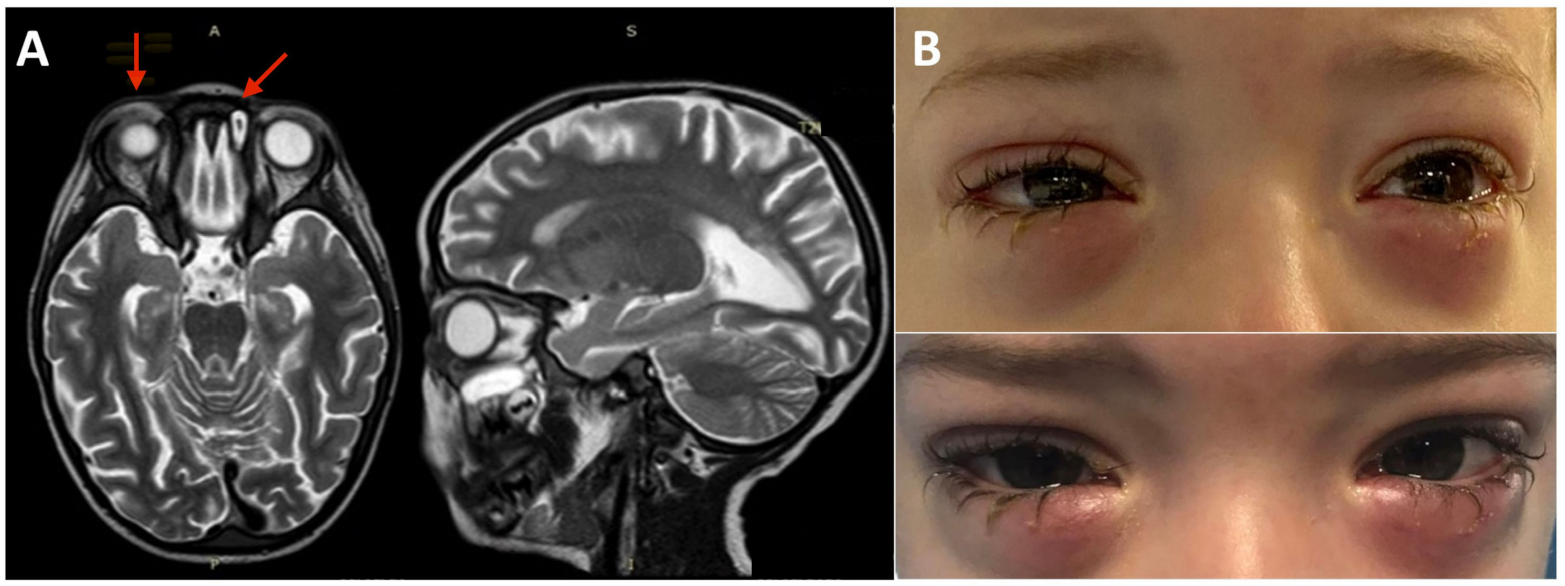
Brain, eye orbit, and paranasal sinus MRI and bilateral lower eyelid edema. (A) MRI of brain, eye orbits, and paranasal sinuses showing bilateral obliteration of the ethmoid-maxillary sinuses, an inflammatory thickening of the periorbital subcutaneous adipose tissue, hypointense in T2 in both orbits, but more evident in the right orbit and brain atrophy and fuzzy T2 hyperintensity of the peritrigonal white matter and corpus callosum. (B) Bilateral lower eyelid edema at admission (top panel) and during the following weeks before rituximab treatment (bottom panel).
